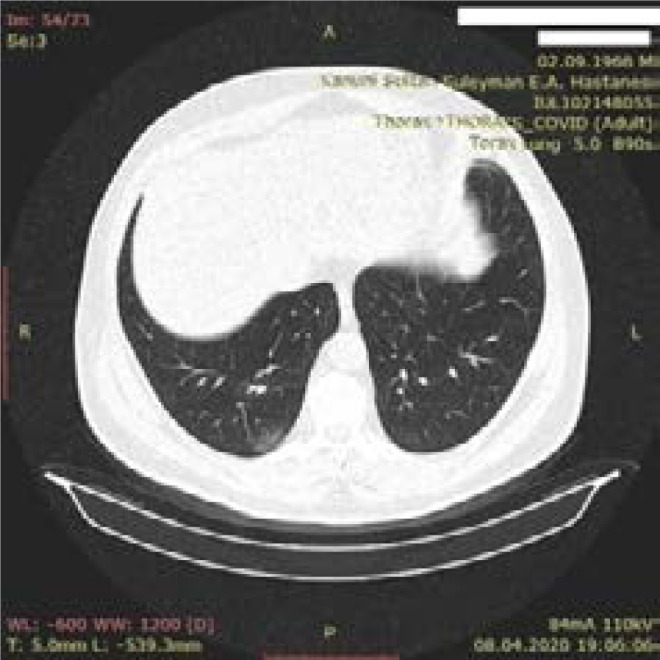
Atypical CT finding - 53-year-old male patient - peripheral focal ground glass density in right lung lower lobe
